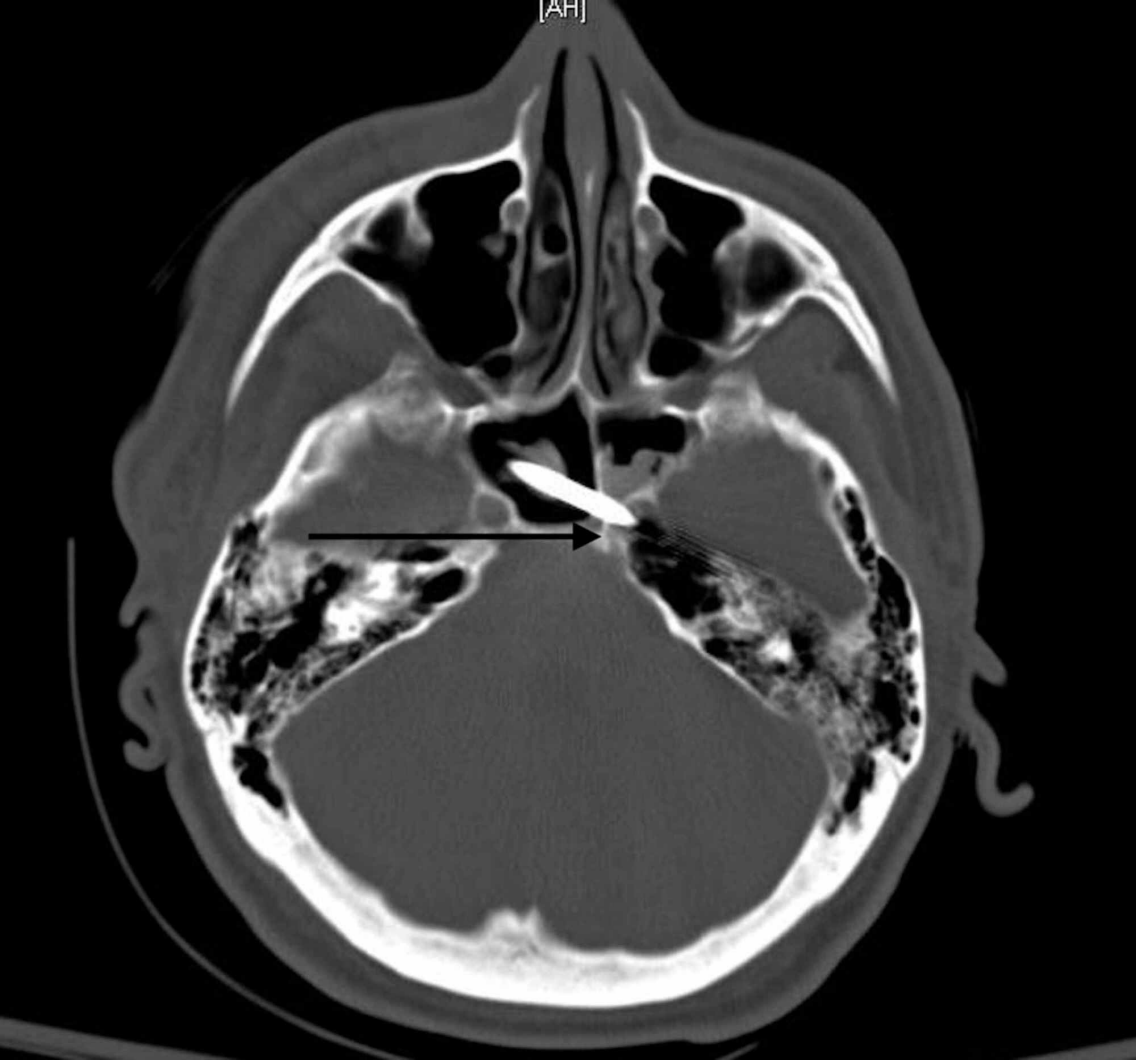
CT of the head The image shows the nail penetrating left petrous carotid canal (black arrow) CT: computed tomography
